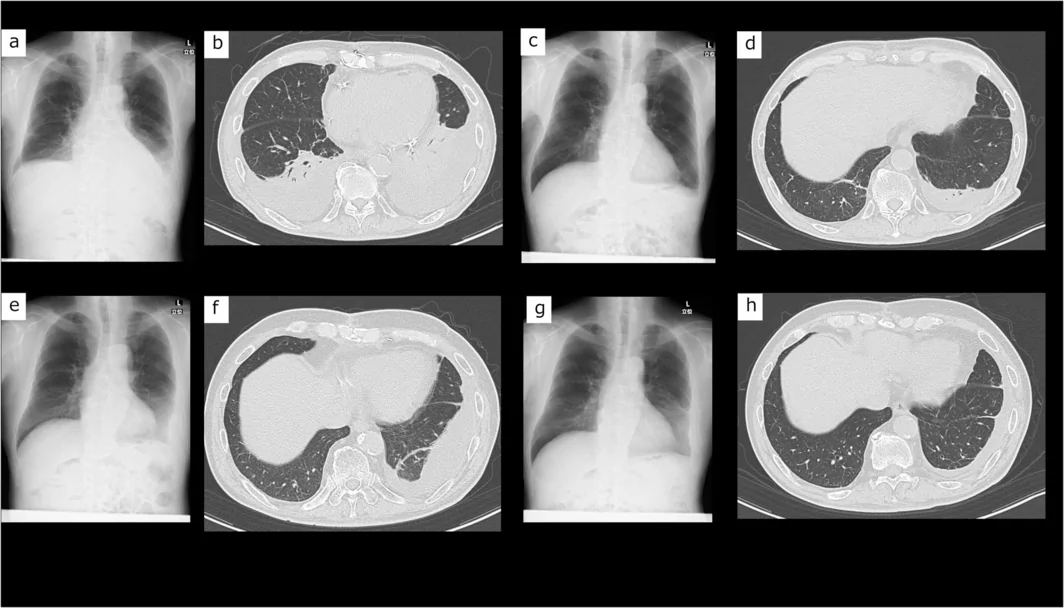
Clinical course of the pleural effusions. (a, b) Chest radiography and computed tomography (CT) on May 7 showing new‐onset bilateral pleural effusions. (c, d) Imaging on May 21 showing partial resolution of the pleural effusions. (e, f) Imaging on August 29 showing worsening of the left effusion. (g, h) Imaging on September 19 showing improvement after ibuprofen treatment.
